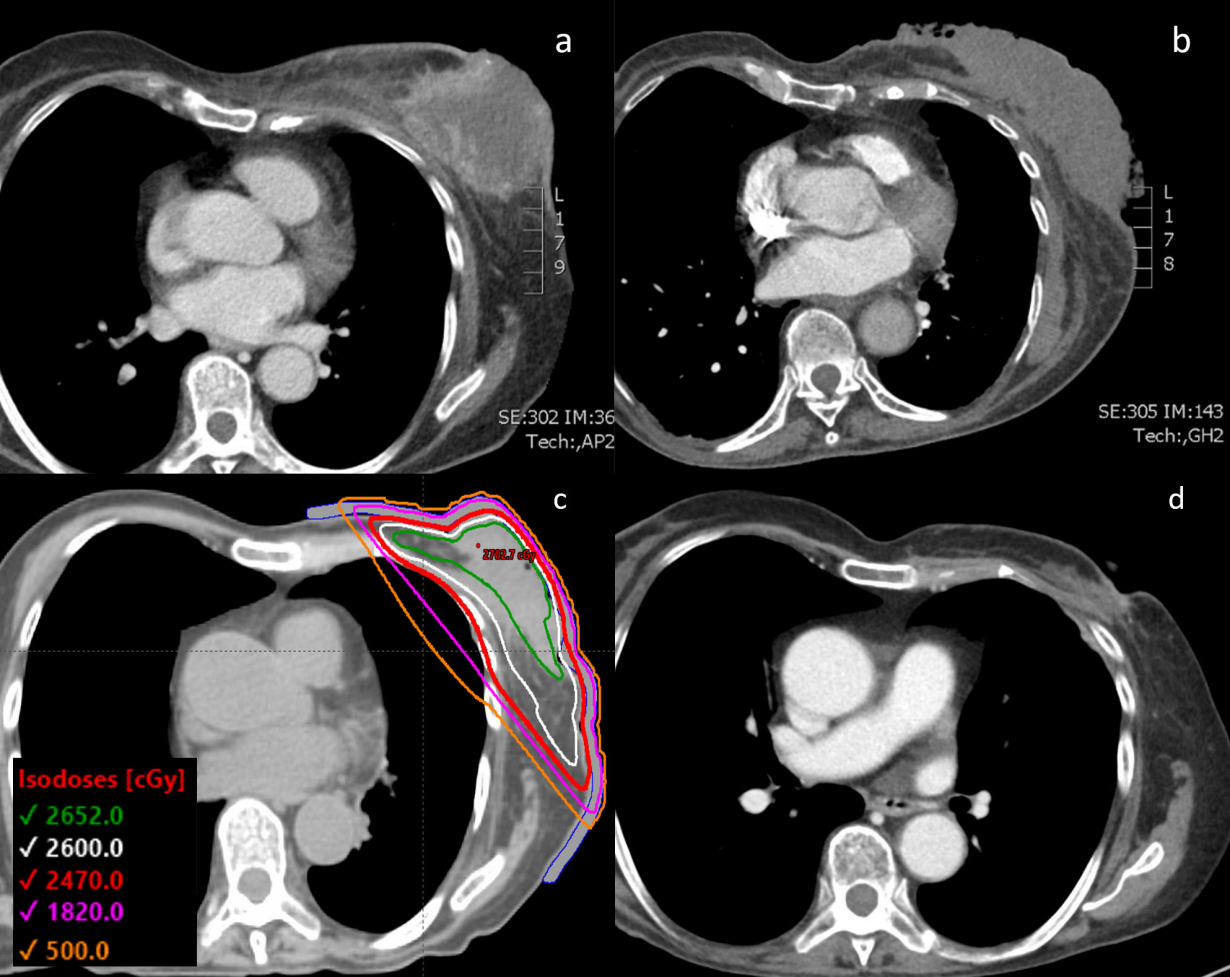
(A) CT chest of the patient with the 12 cm diameter left-sided fungating breast mass shown at the time of initial diagnosis. (B) Tumor visualized after progressing through initial line of paclitaxel chemotherapy and right before initiating FAST-Forward radiation therapy. (C) Radiotherapy plan with prescribed dose of 26 Gy in 5 fractions with a 0.5 cm bolus. (D) CT chest showing the 1.8 cm tumor 3.5 months after completion of radiation therapy and starting doxorubicin chemotherapy.
